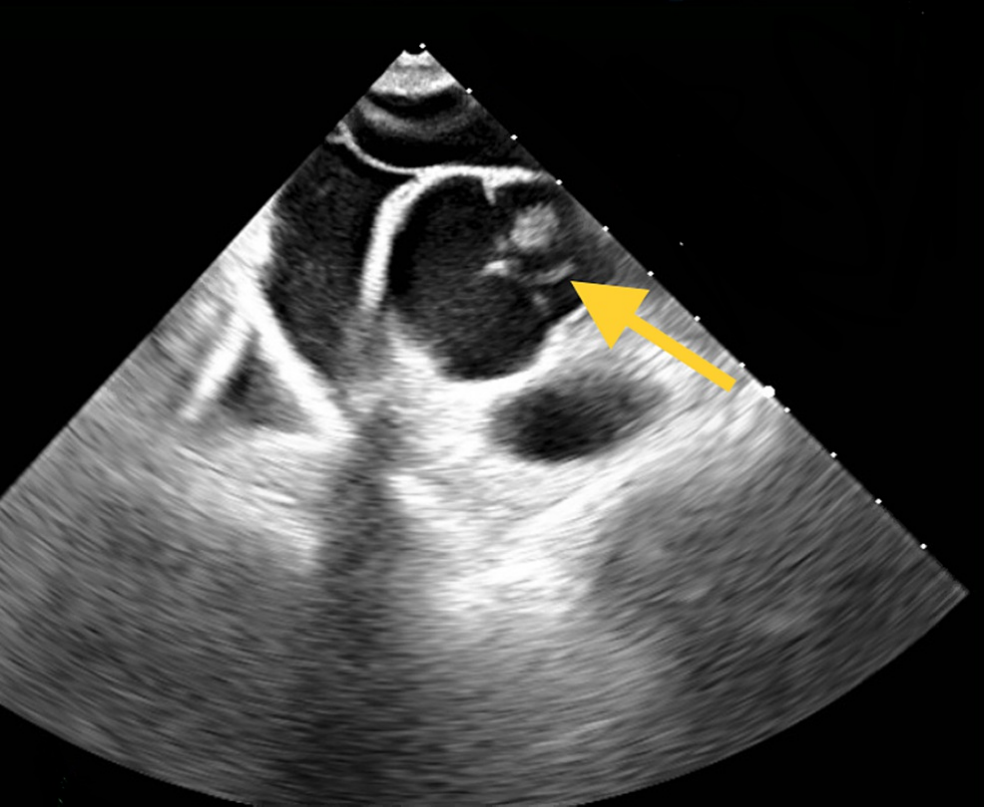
TEE showing 7-mm presumed fibroelastoma present on the aortic valve (yellow arrow) TEE: transesophageal echocardiogram
